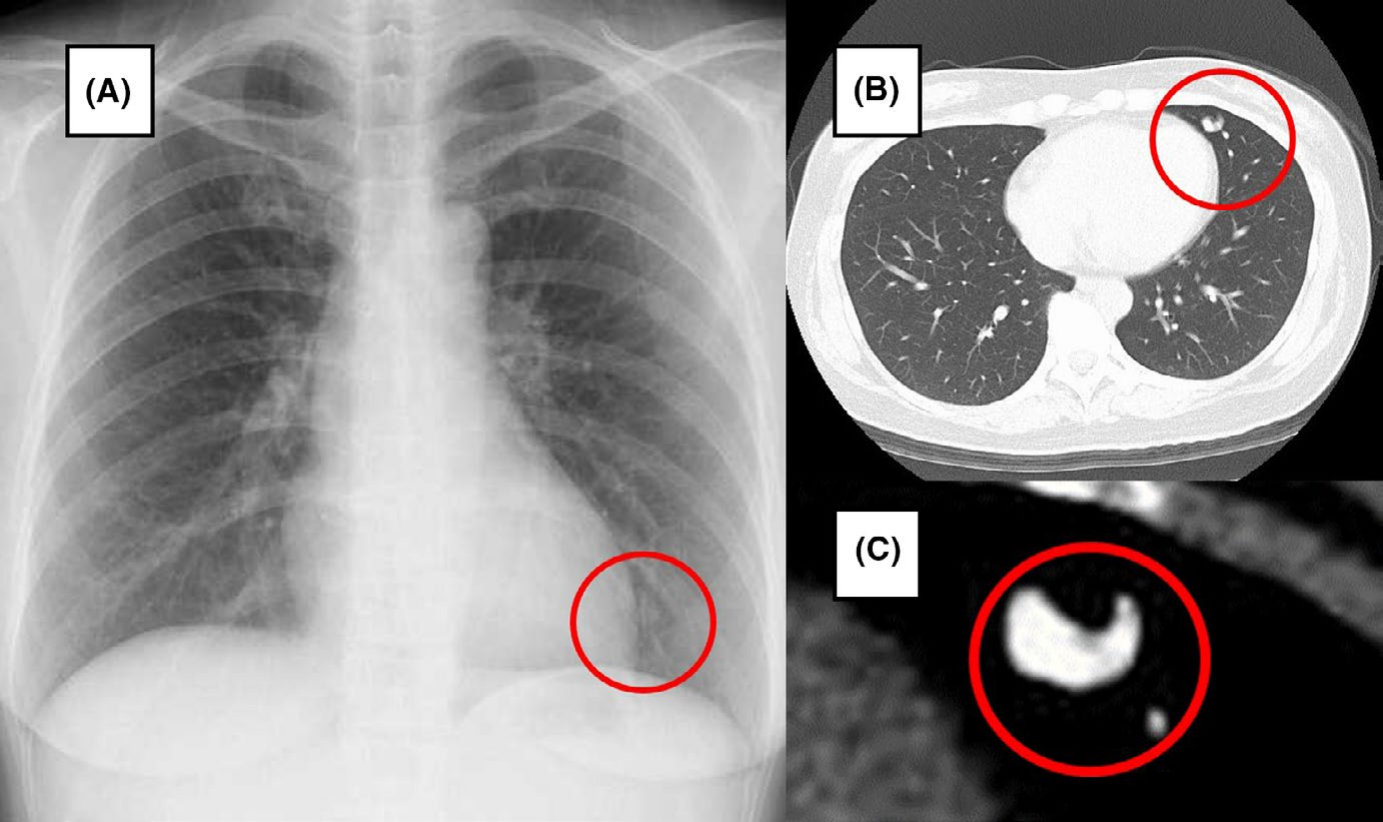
Chest X‐ray (A) and the enhanced computed tomography (B,C) showed pulmonary AVF which was 1.5 cm in the peripheral left superior lingular segment which was contrasted (C, inside red circle)
